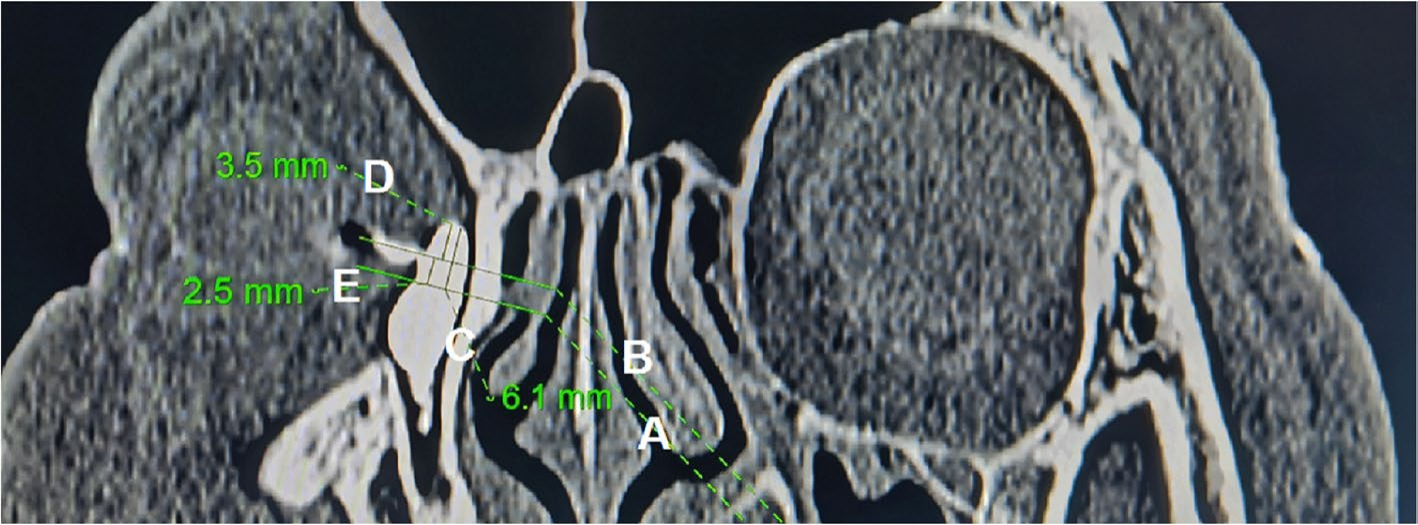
Measurements in the long axis of a large lacrimal sac on computed tomographic dacryocystography (CT-DCG) parasagittal images. A, a line drew from the middle turbinate axilla (MTA) to the long axis of the sac at right angles. B, a line drawn through the common canaliculus (CC) to the long axis of the sac at right angles. C, the height of the sac above the MTA. D, the height of the sac above the CC. E, the distance from the MTA to the CC
